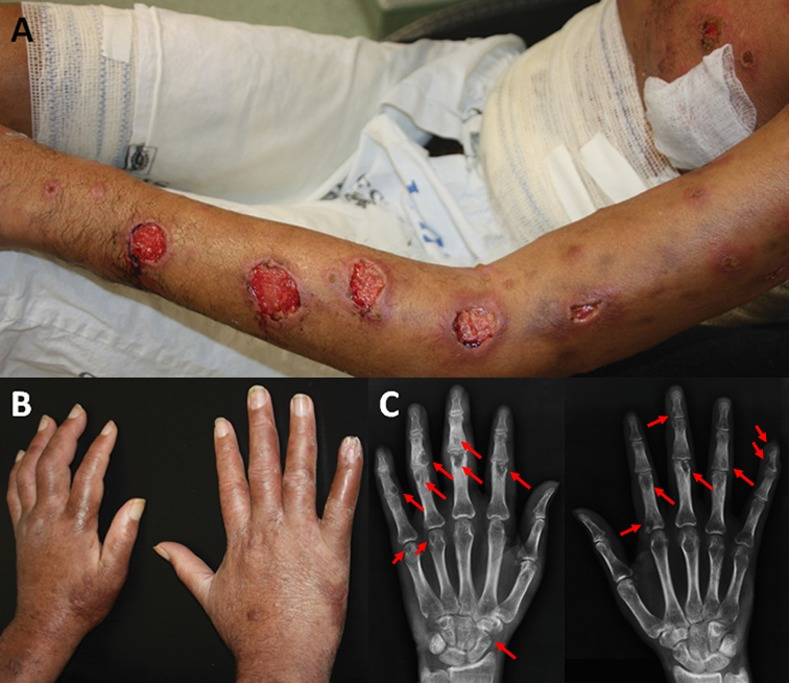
Multifocal form (case 29). A) Extensive ulcerated sporotrichosis lesions on the left upper limb and trunk. B) Hands with edema, more noticeable to the right (clinical images were inverted to correspond to the radiographs). C) Radiography—Multiple lytic lesions and bone erosions in both hands (arrows). (Source: A-B—Images by Dr. Marcelo Rosandiski Lyra; C–Service of Image of the INI).
